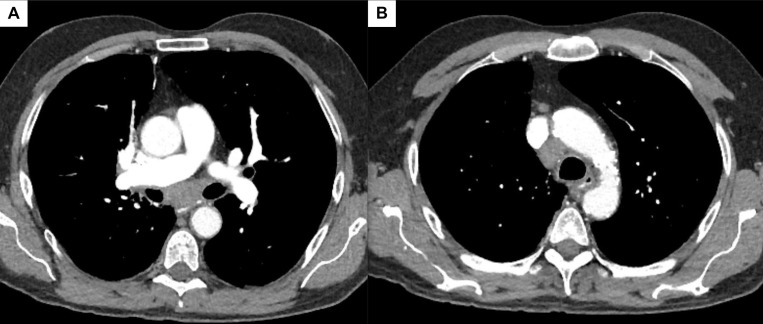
Figure 1: CT scan of the chest with contrast in the mediastinal window and transverse plane showing an enlarged (A) subcarinal and (B) lower right paratracheal lymph node.
